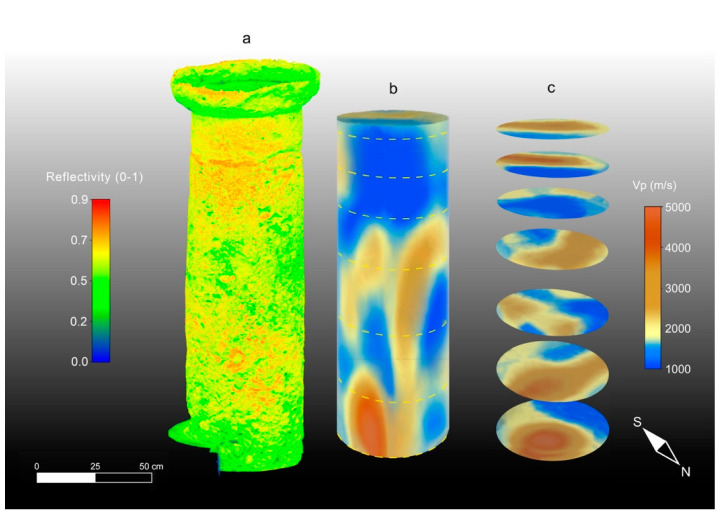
High-resolution 3D models of column 2. (a) TLS reflectivity model; (b) ultrasonic tomography; (c) tomographic slices.
